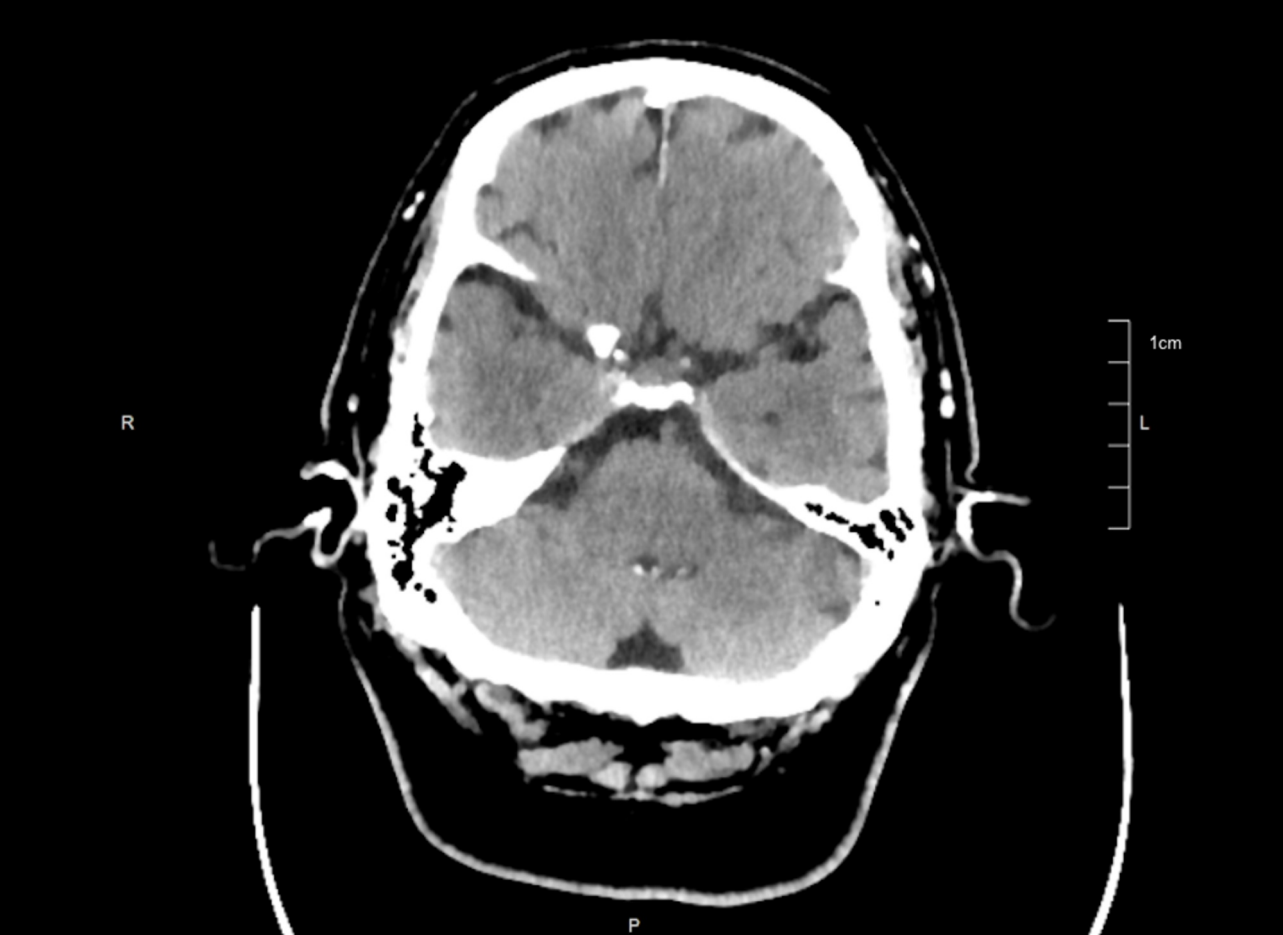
Computed tomography of the head showing no evidence of acute intracranial pathology
